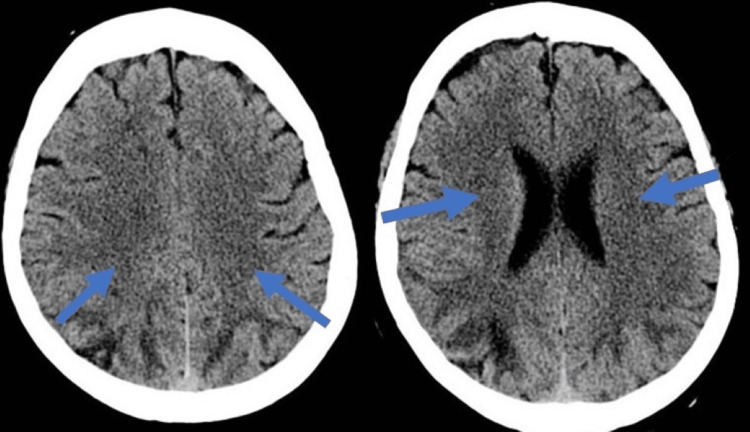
CT brain showing diffuse edema in the periventricular region of both cerebral hemispheres
